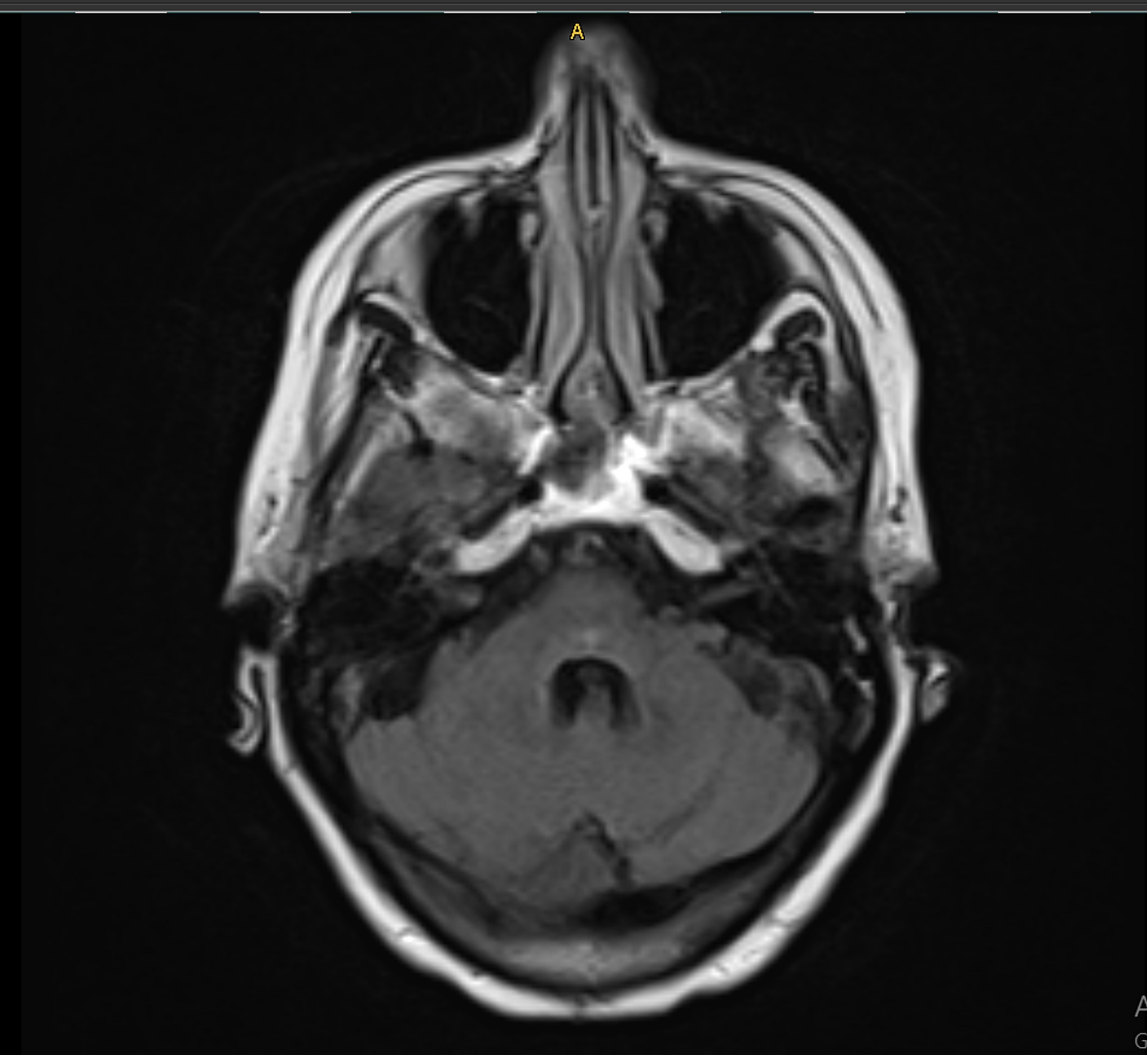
T2 FLAIR post-treatment. FLAIR, fluid-attenuated inversion recovery
